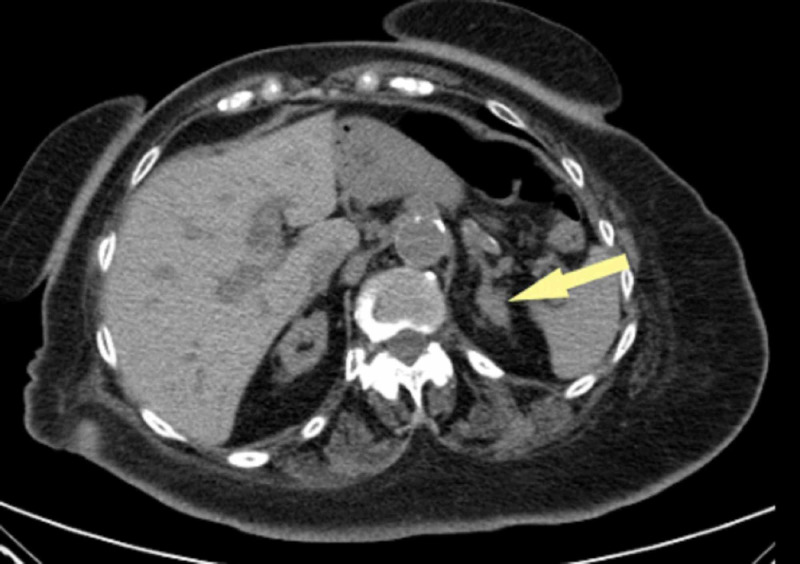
CT scan of the abdomen and pelvis without contrast showing a low-attenuation mass in the left adrenal gland measuring 15.8 mm. An atrophic kidney is seen on the right side.
